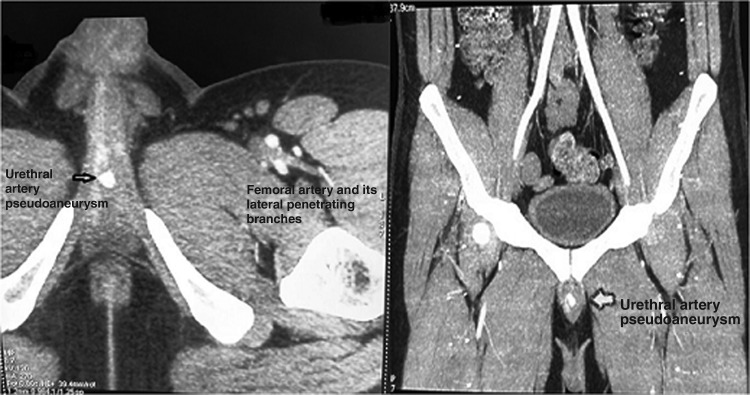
CT angiography images (axial, sagital cuts) showing pseudoaneurysm in the region of penile urethra, which was confirmed to be arising from bulbourethral artery in dynamic images.
